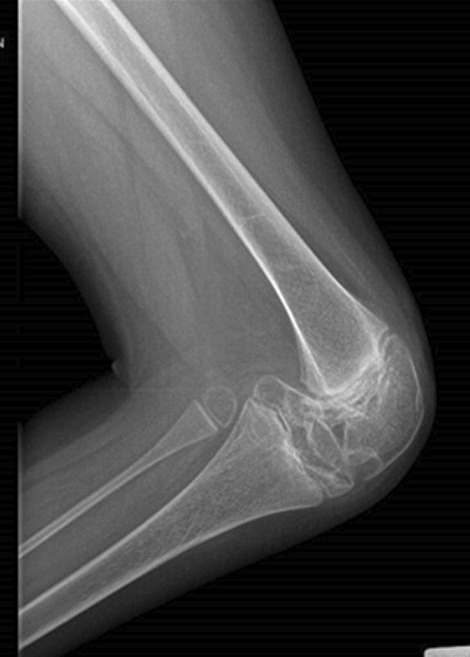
Pt. 2 X-ray at age 11y 6m. Knee radiograph showing important irregularity of the femoral condyles and proximal tibia
